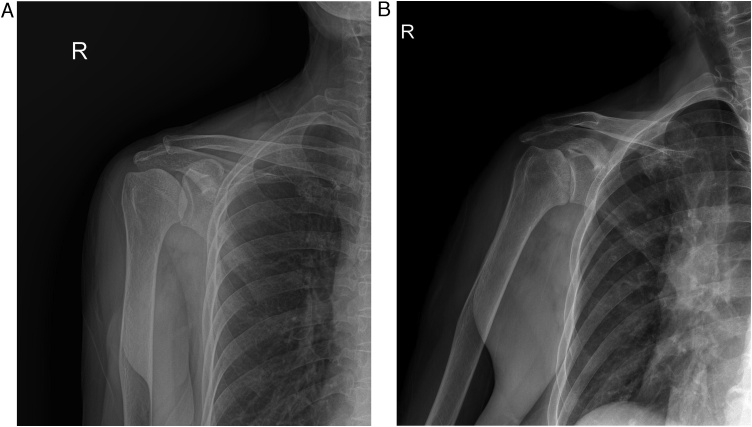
Right shoulder plain anteroposterior (AP) radiograph (a). Rapid progression of joint destruction seen at the 4-week follow-up in the AP view (b).
